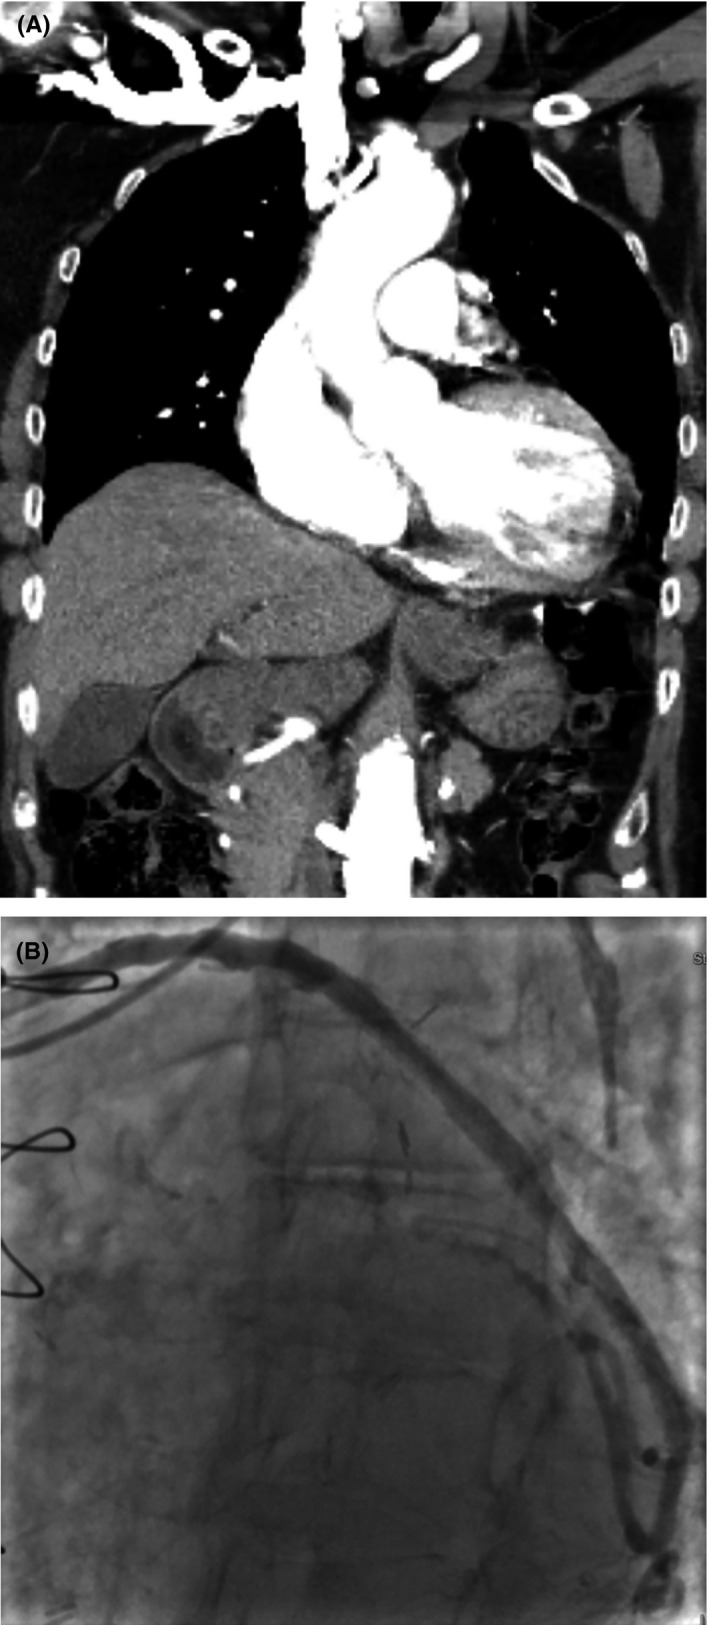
A, Contrast CT angiogram showing no aneurysmal dilation 6 y ago. B: Coronary angiogram of SVG to OM showing no significant aneurysmal dilation 6 y ago
